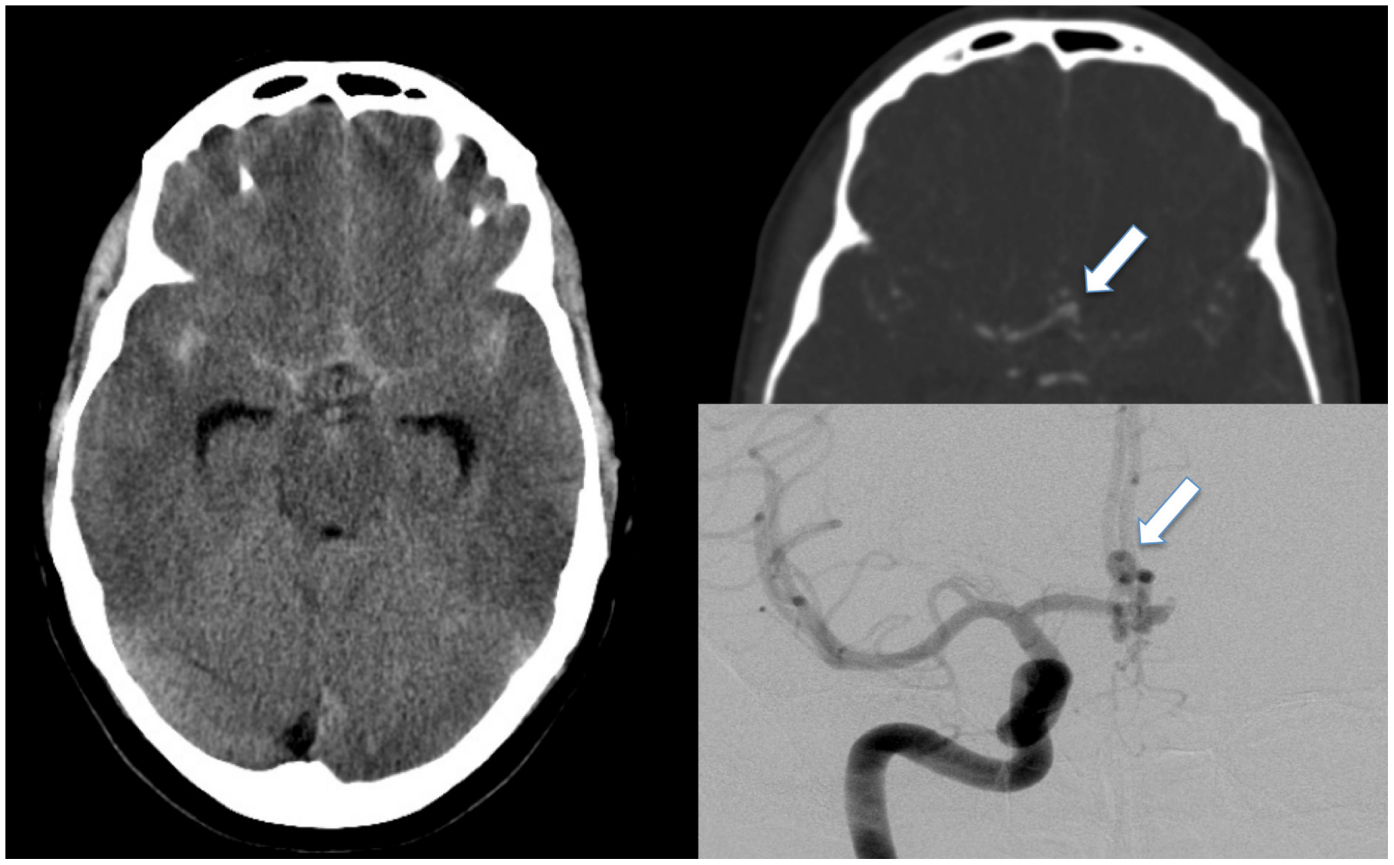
45 year old woman presenting with acute thunderclap headache. Inital CT showed subarachnoid hemorrhage. Contrast enhanced CTA revealed an arteria cerebri anterior aneurysm with a diameter of 3(DSA).
